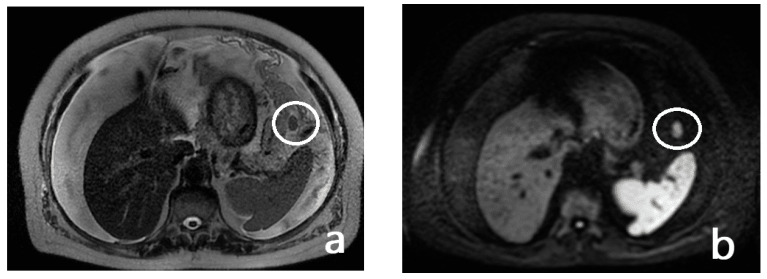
A 73-year-old man with a diagnosis of signet-cell gastric adenocarcinoma located in the greater curvature. T2w axial (a) and DWI b1000 axial (b) MR images of the upper abdomen show tumoral implants (white circles) in the gastrosplenic ligament. Note also the presence of free peritoneal fluid.
